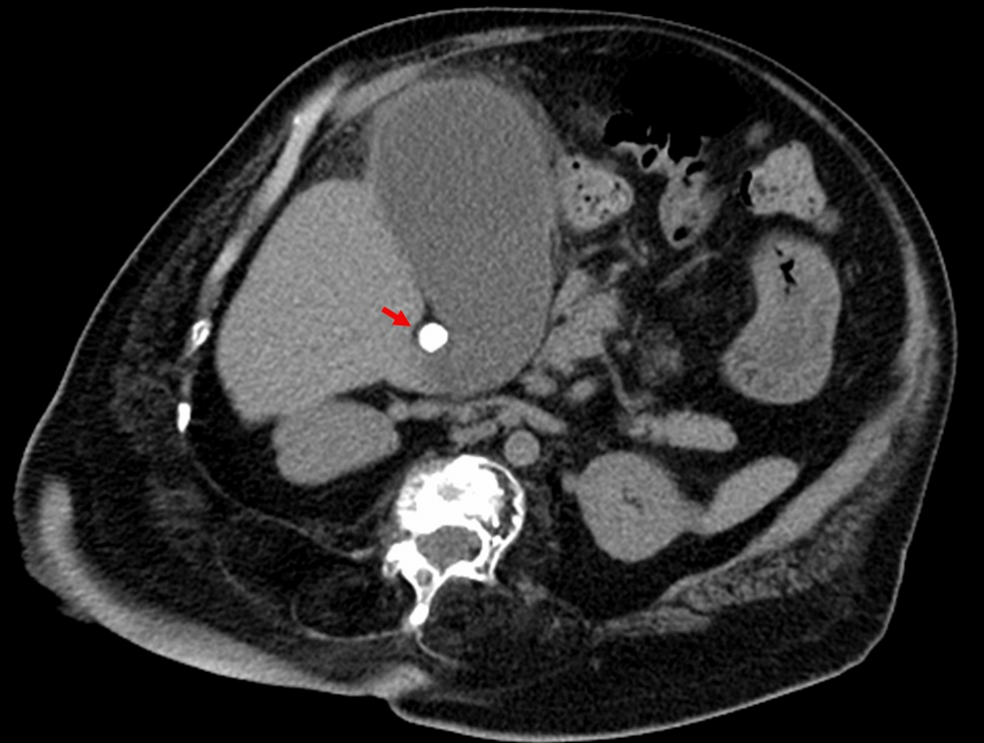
Computed tomography image on admission The gallbladder was swollen and its wall was thickened. An impacted stone can be seen at the gallbladder neck (arrow).
